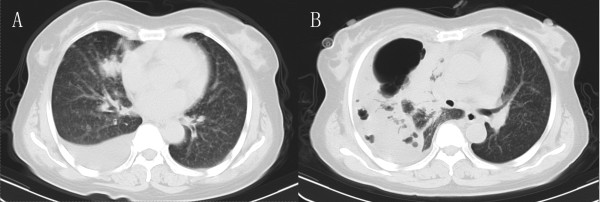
Chest CT scans of preoperative and postoperative. A: A chest CT scan showed a 20 × 25 × 20 mm mass on the right middle lobe as well as an amount of pleural effusion on admission. B: An emergent chest CT scan showed a huge gas cavity and liquid located in the up right chest cavity.
